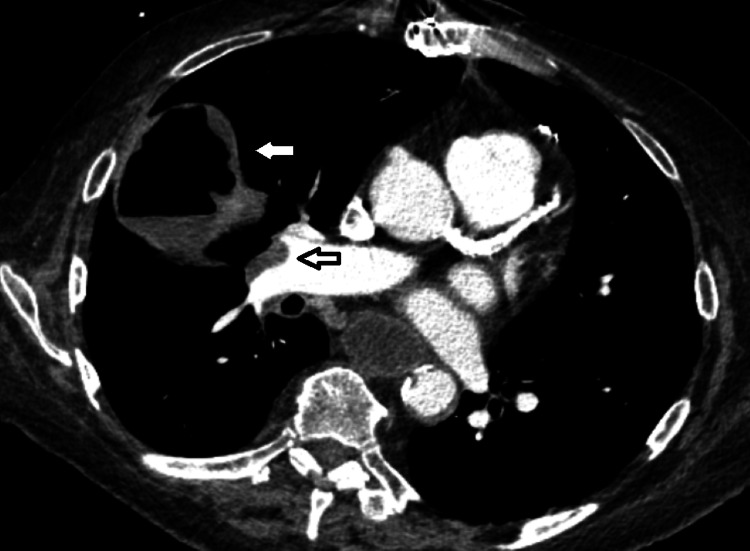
Mediastinal window CT images demonstrating a cavitary lesion with an air–fluid level in the right middle lobe abutting the pleura (white arrow) and partial visualization of a pulmonary embolus within the right pulmonary artery (black arrow).
